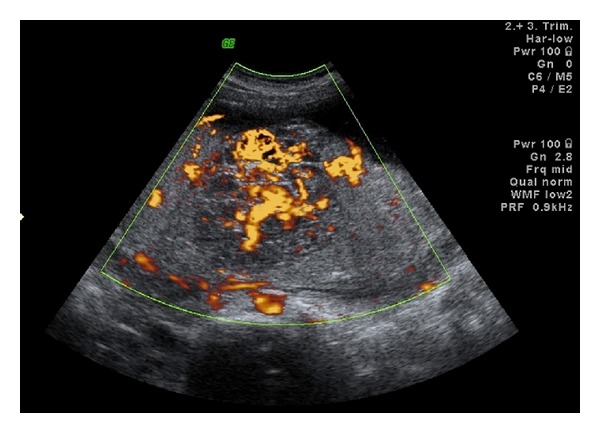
The power Doppler ultrasonography of the placental angioma of Case 1 showing rich vascular supply within.
